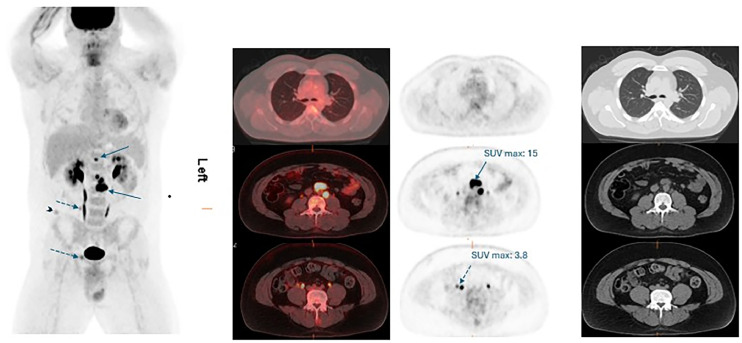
Coronal maximum intensity projection (MIP) FDG PET image obtained for staging following radical orchiectomy for testicular embryonal carcinoma. Markedly hypermetabolic retroperitoneal lymph nodes (SUV max: 15) consistent with nodal metastatic disease are identified (solid arrows). Moderately hypermetabolic right common iliac and right external iliac lymph nodes (dashed arrows) were considered indeterminate, with differential considerations including reactive/inflammatory changes versus nodal metastasis. Importantly, no suspicious FDG uptake is identified in the chest, and the lungs are clear without evidence of pulmonary metastatic disease at this time. Focal moderate FDG uptake projecting above the right iliac crest corresponds to a benign cutaneous inflammatory uptake (blue arrowhead), The remainder of the body shows no evidence of distant metastatic disease. Physiologic radiotracer activity is noted in the brain, myocardium, kidneys, and urinary bladder.
